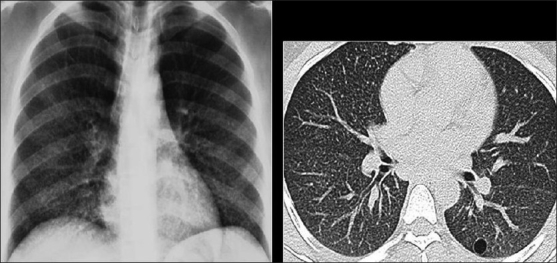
Pneumocystis carinii* pneumonia atypical features. Chest X-ray shows finely granular/miliary appearance better depicted on high-resolution computed tomography
